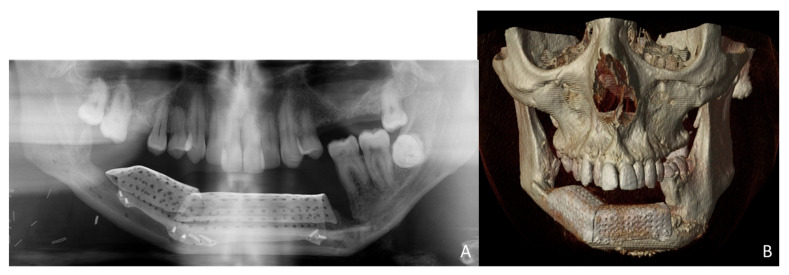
Postoperative images after 3D reconstruction with CAD/CAM titanium mesh and iliac crest graft. (A) Panoramic radiograph showing the vertical reconstruction of the symphysis and both mandibular bodies with the iliac crest graft. (B) CT Scan with three-dimensional reconstruction of the mandible.
